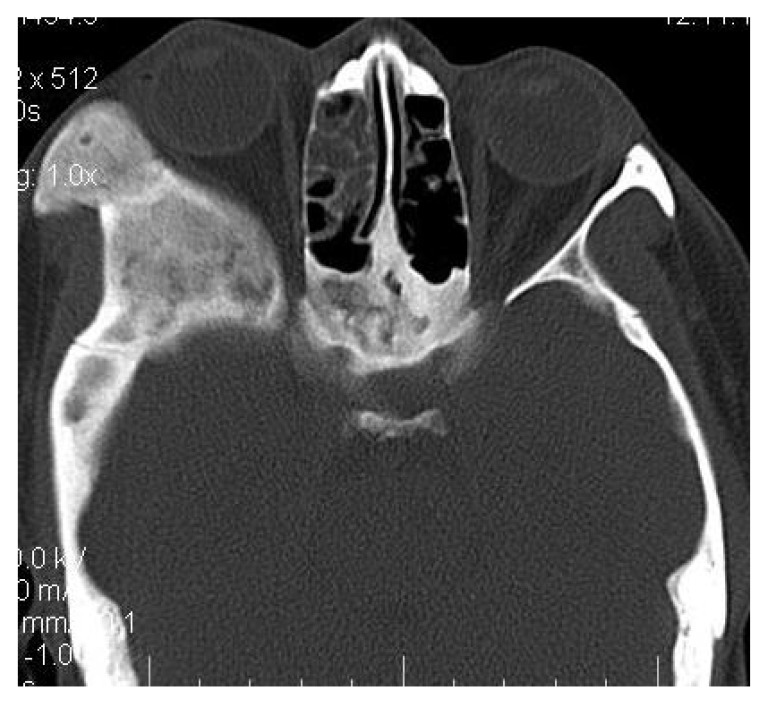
The Axial CT section revealing the involvement of the lateral wall of right orbit, right Zygomatic arch, zygoma, right pterygoid region with right craniaum. Further there was evidence of narrowing of the of the optic canal of right side.
